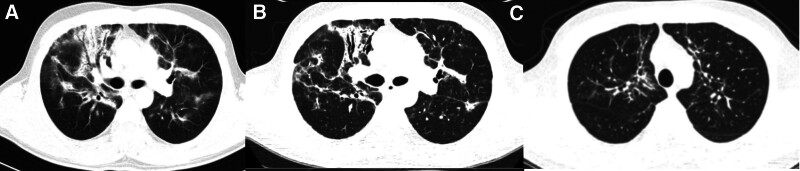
Chest CT scans showing the progression and resolution of findings over time. (A) July 2022: Bilateral ground-glass areas and interstitial opacities. (B) August 2022: Resolution of bilateral interstitial opacities and lobar consolidation. (C) February 2024: Development of multiple pulmonary nodules. CT = computed tomography.
